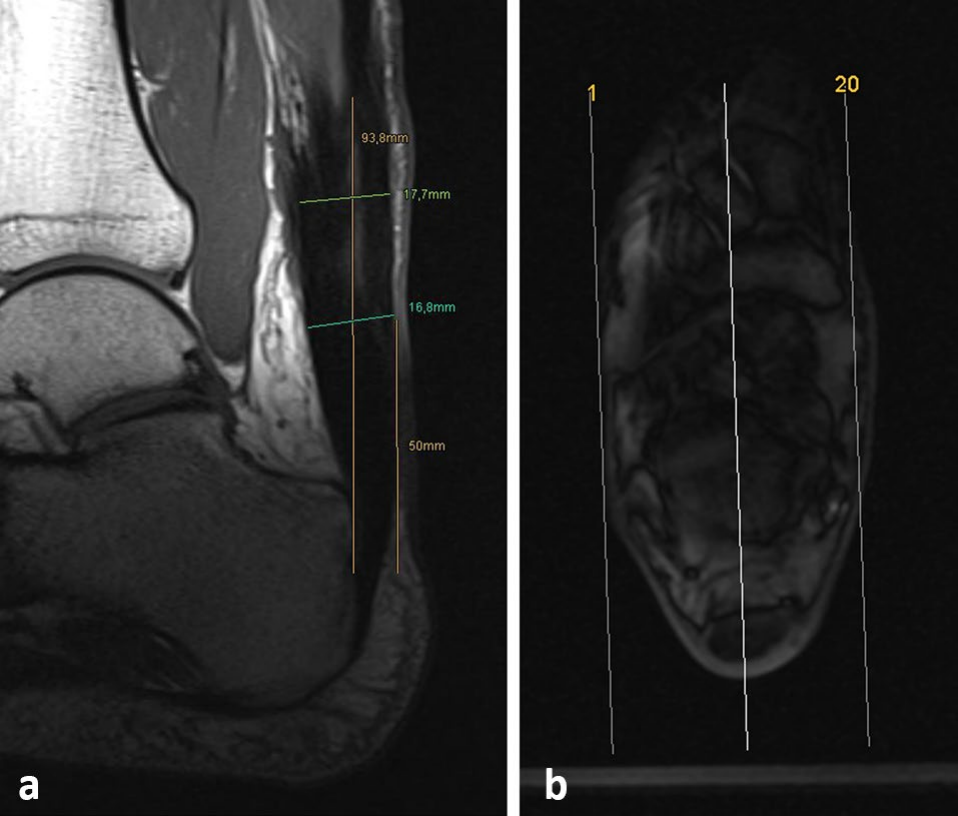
MRI measuring technique. Measuring the length of the tendon from the insertion to the musculotendinous junction, the diameter of the tendon at its most bulky point and 5 cm above the insertion (a) using the localizer in the second layer to determine the tendon midline (b)
